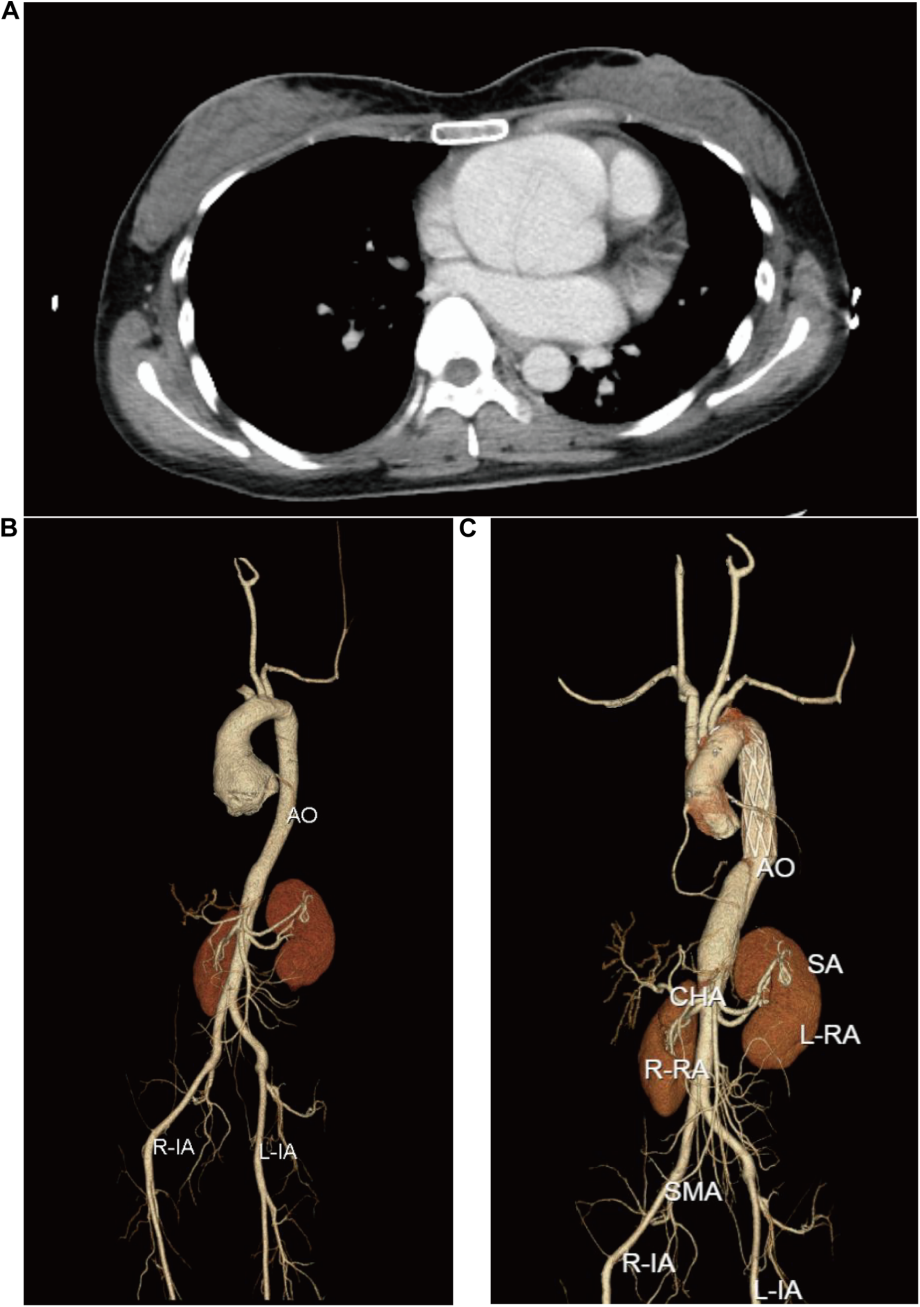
Cardiovascular disorders nin the proband. (A) CT image of the aortic root. (B) and (C) depict the multiplanar reconstructions of the aorta before and after David and Sun's procedure, respectively.
